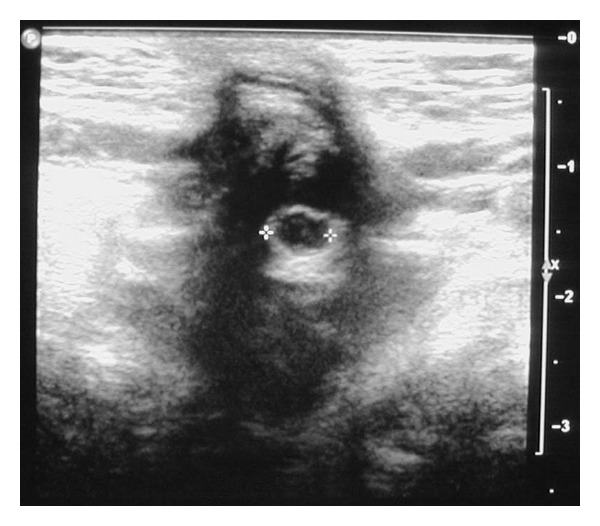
Umbilical cyst visualized by ultrasound through the anterior abdominal wall.
